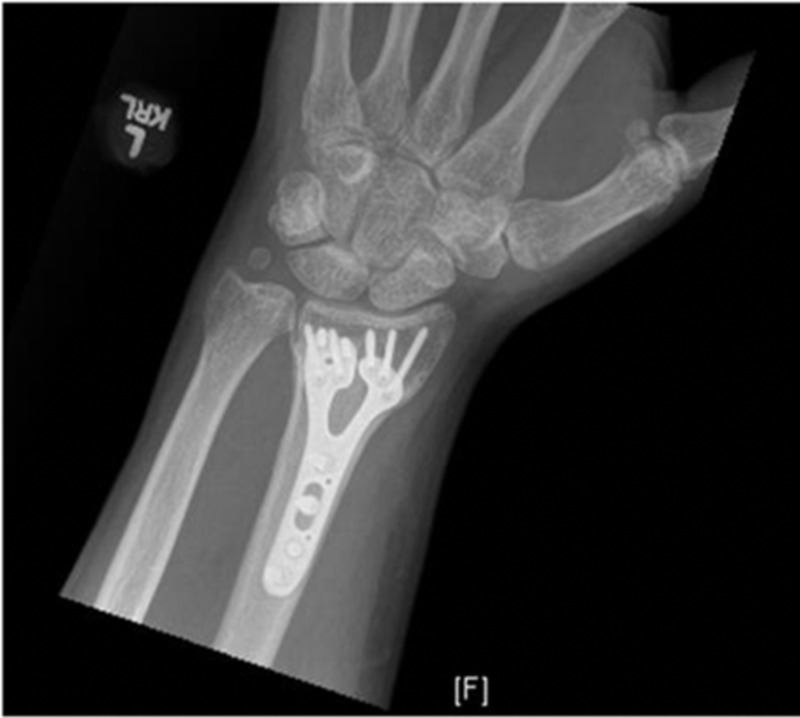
Anterior-posterior (AP) X-ray of the left wrist showing volar plate fixation with a healed distal radius fracture and small displaced ulnar styloid fracture which appears stable from previous X-rays
